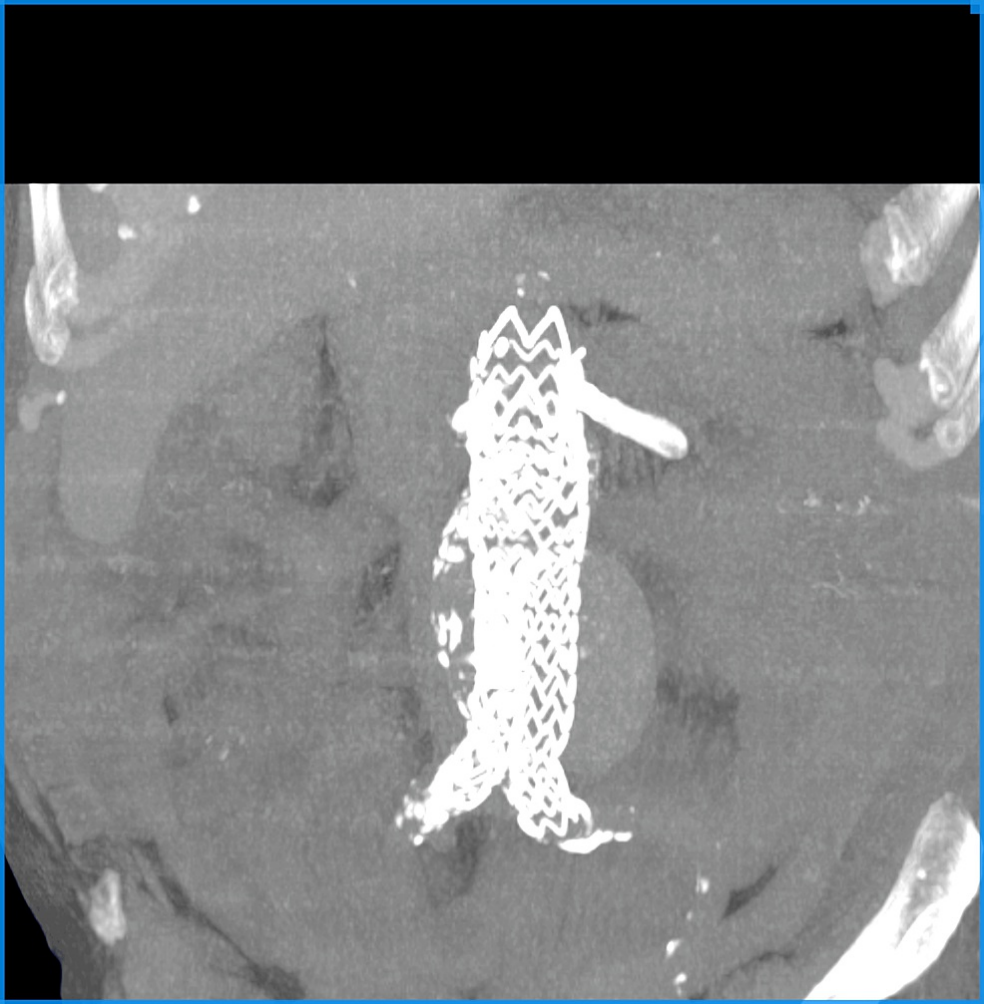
Case 2: CT without contrast showing the stent graft with two chimneys. CT, computed tomography
